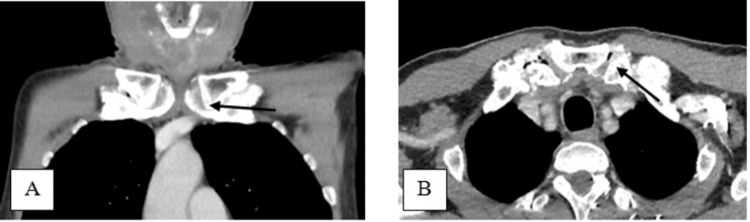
Coronal (A) and axial view (B) Contrasted CT scan of the neck showing periarticular calcification at the bilateral sternoclavicular joint (black arrow)
